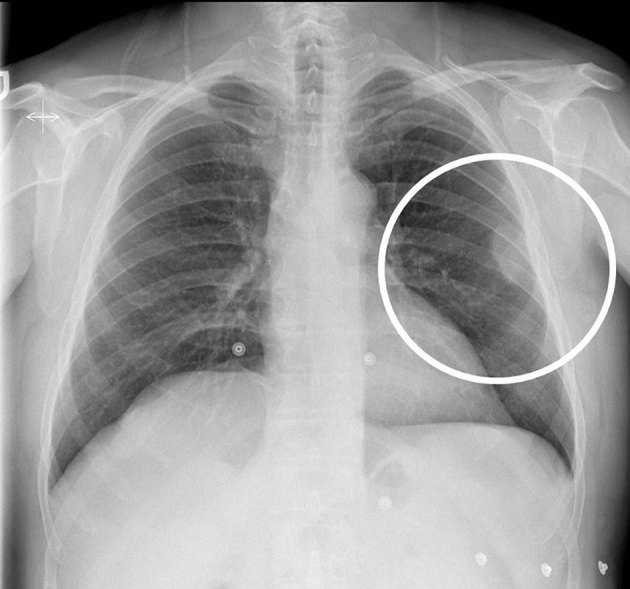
Chest X-ray showing a rounded subpleural mass in the upper left hemithorax (circle). No rib invasion is seen.
